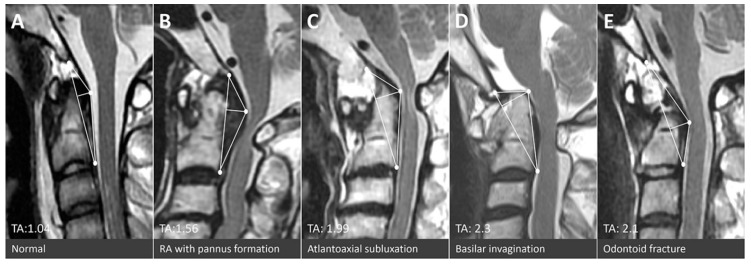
Mid-sagittal T2 weighted magnetic resonance images of patients with (A) a normal CVJ, (B) rheumatoid arthritis with pannus formation, (C) atlantoaxial subluxation, (D) basilar invagination, and (E) odontoid fracture.
